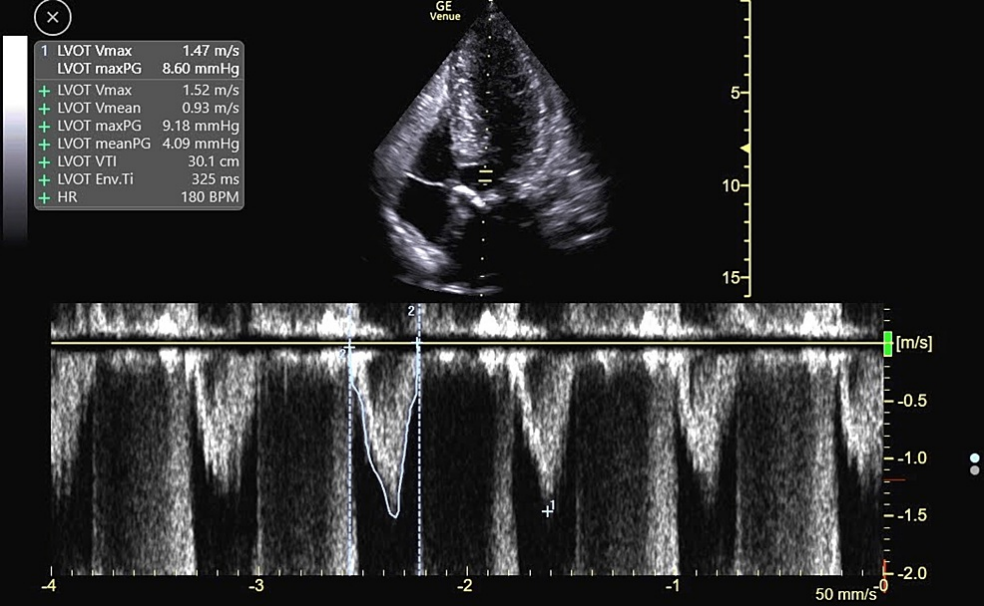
Pulsed-wave Doppler of the LV outflow tract revealing a late-peaking velocity envelope. Also, note the concomitant aortic regurgitation velocity profile during diastole. LV: Left ventricle.
